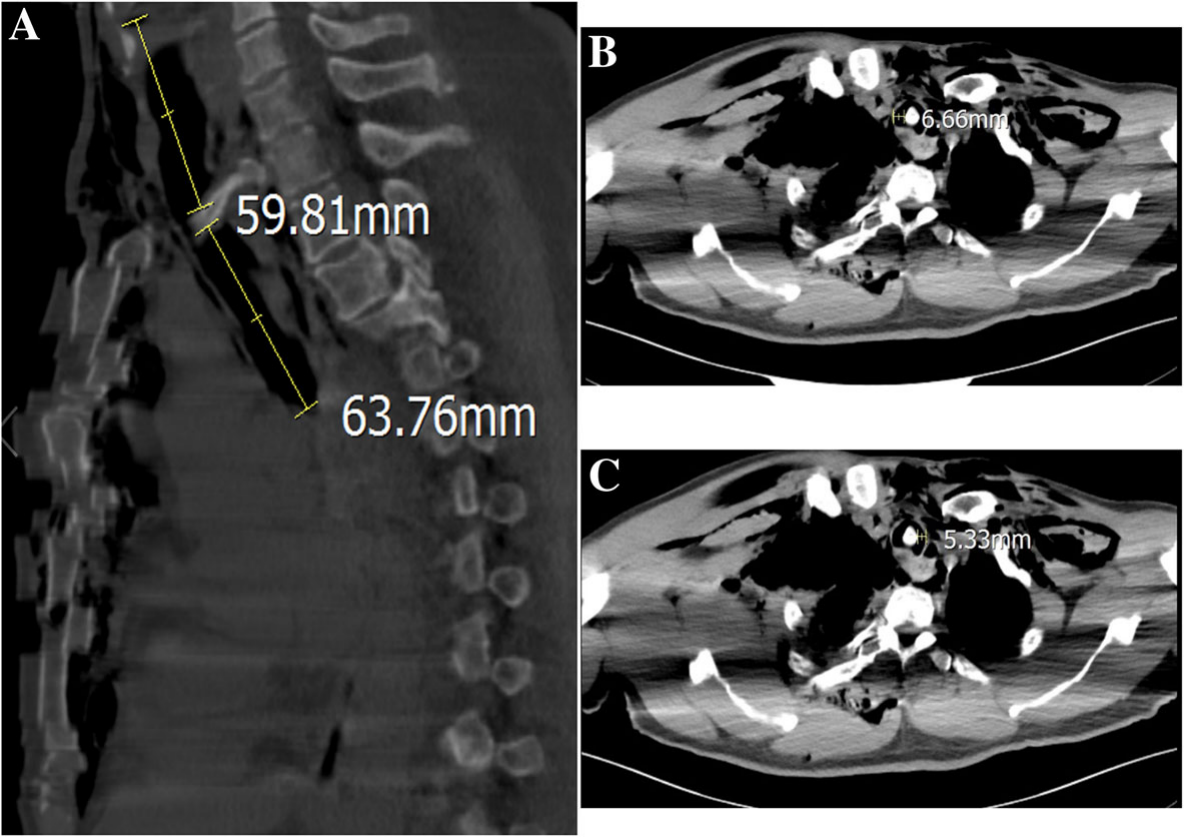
Preoperative evaluation of Tracheobronchial lacerations by a high-resolution CT. a Sagittal CT image of the chest showing the posterior tracheal wall laceration up to 59.81 mm below the glottis and 63.76 mm above the carina. b, c Axial CT image of the chest showing the bone shadow in the trachea; the residual largest cavity of the trachea on the left was 5.33 mm in diameter and 6.66 mm on the right
